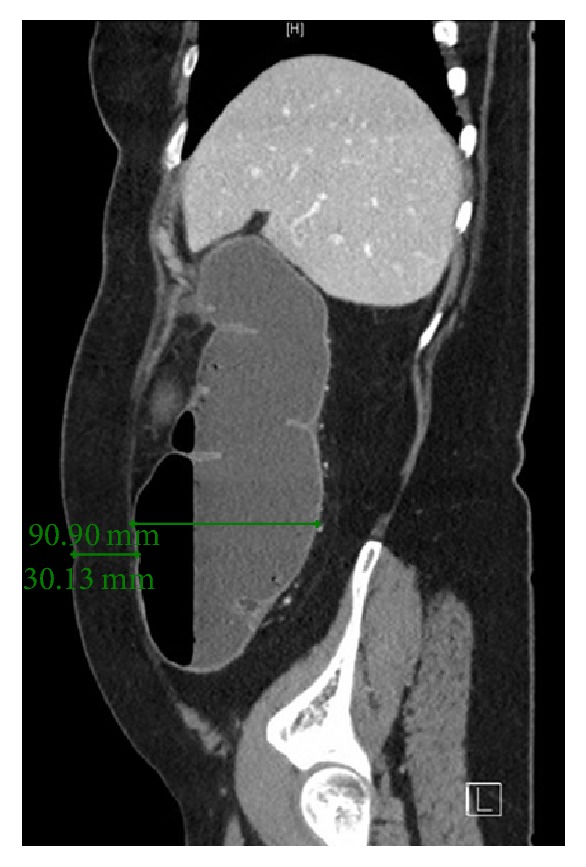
Sagittal CT showing caecum measured from the anterior abdominal wall.
